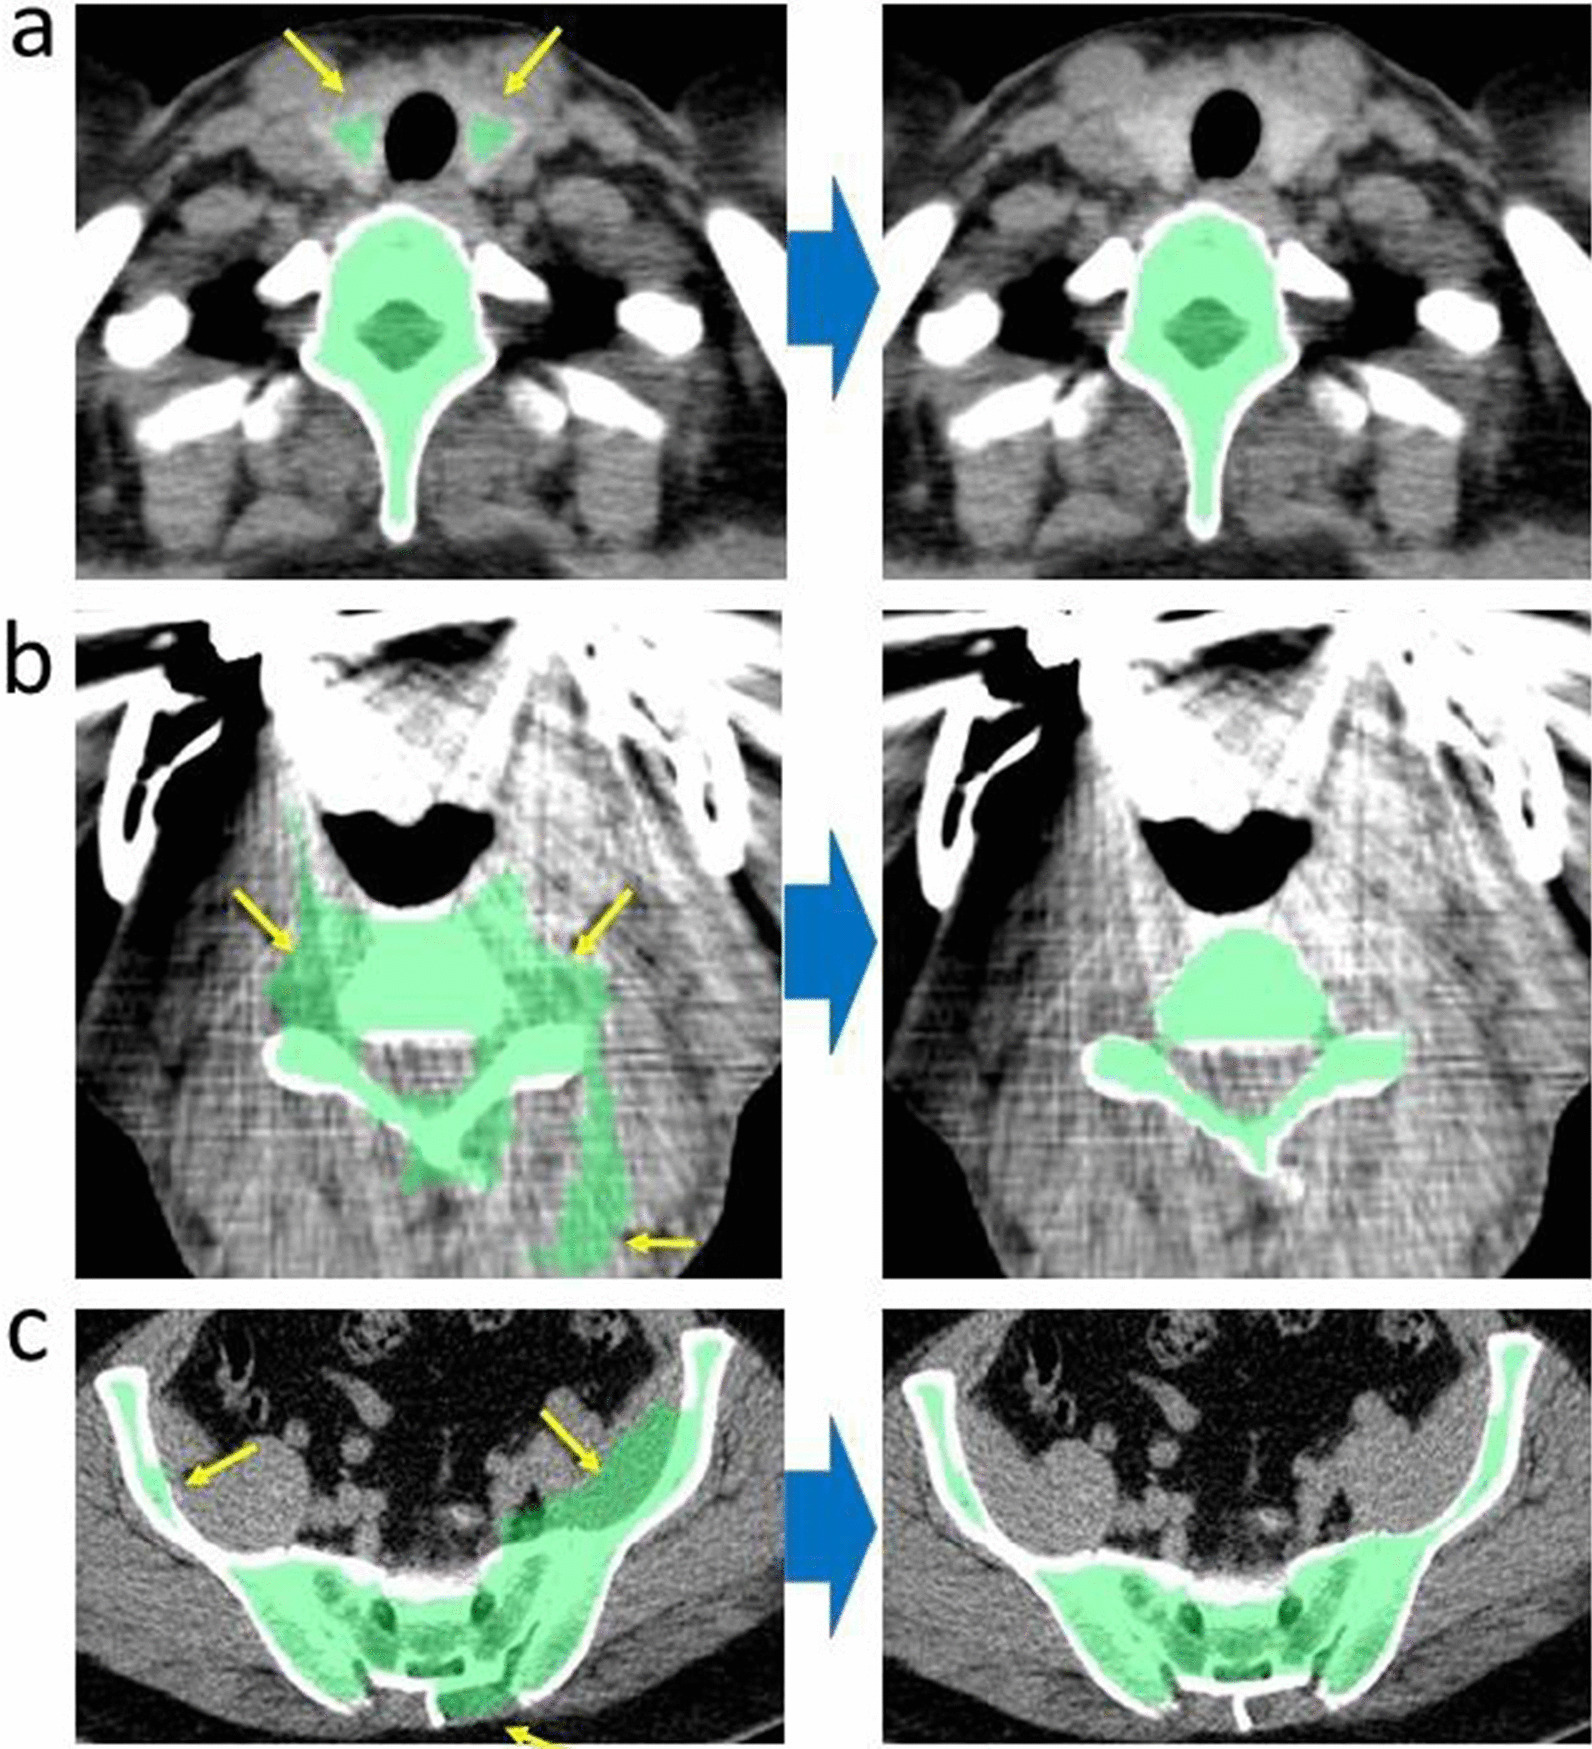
Addition of manual removal of the extracted extramedullary region due to high CT value, thyroid gland (a), metal for dental treatment (b). Images of a case where the pelvic muscles extracted continuously with the pelvic bones were manually removed (c). No obvious artifacts were visible
